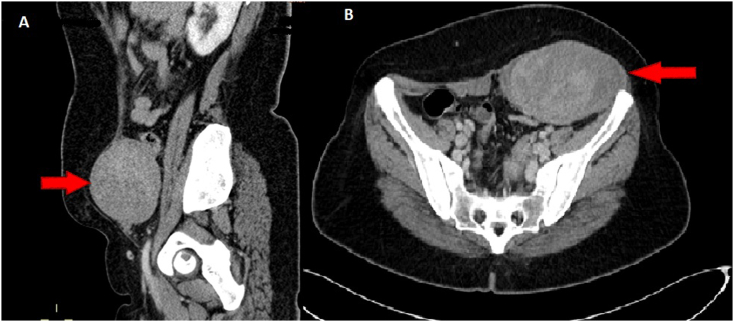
Abdominal CT scan showing a mass occupying the left anterolateral abdominal wall reaching the left iliac crest A: Coronal section B: Axial section.
